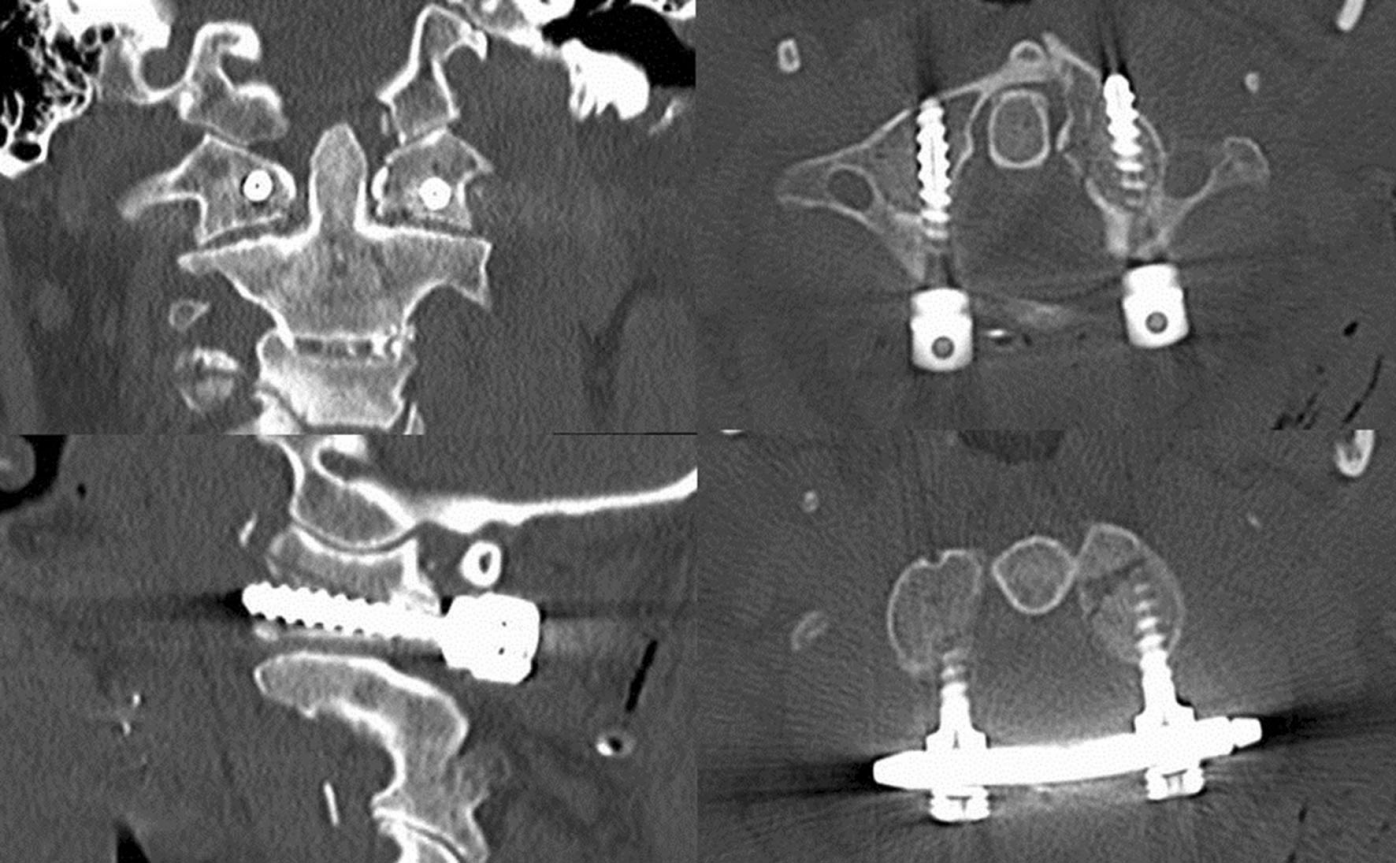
Coronal (*top left*), sagittal (*bottom left*) and transversal (*right*) CT images of patient 1 after ring osteosynthesis. The left lateral mass of C1 is reduced and fixed by monoaxial screws and a rod
